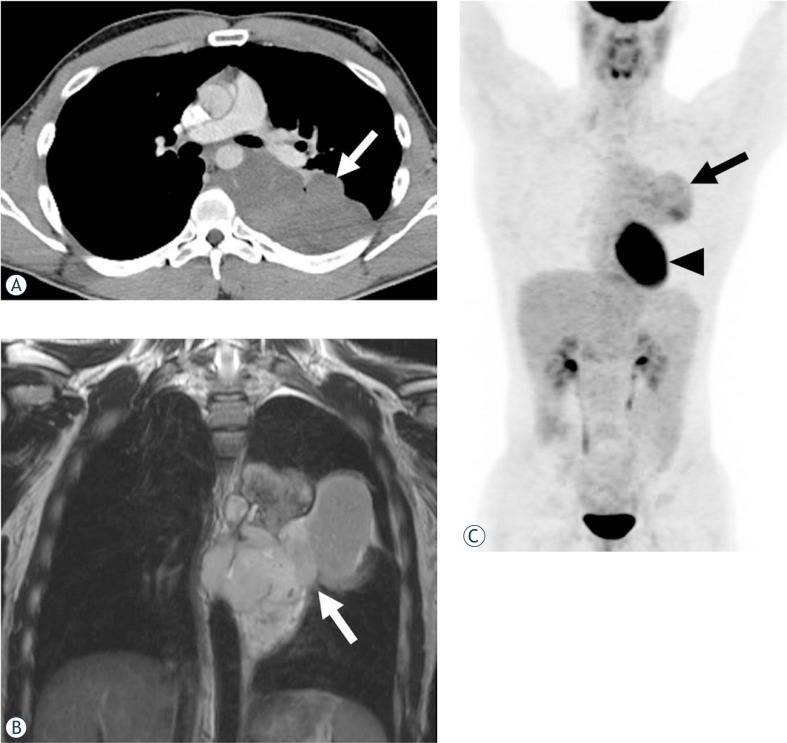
A 31-year-old man with mediastinal malignant peripheral nerve sheath tumor. A. Axial contrast-enhanced CT (CECT) shows a low attenuating mildly enhancing posterior mediastinal mass (arrow) displacing the aorta, azygous vein and esophagus. The mass encompasses more than 50% circumference of the descending thoracic aorta. B. Coronal T2-weighted MR image shows a heterogeneous lobulated hyperintense mass (arrow). C. Coronal maximum intensity projection (MIP) PET image shows a moderately fluorodeoxyglucose (FDG) avid mediastinal mass (arrow, standardized uptake value [SUV]_max_ 5.2). Physiologic activity is noted in the heart (arrowhead).
